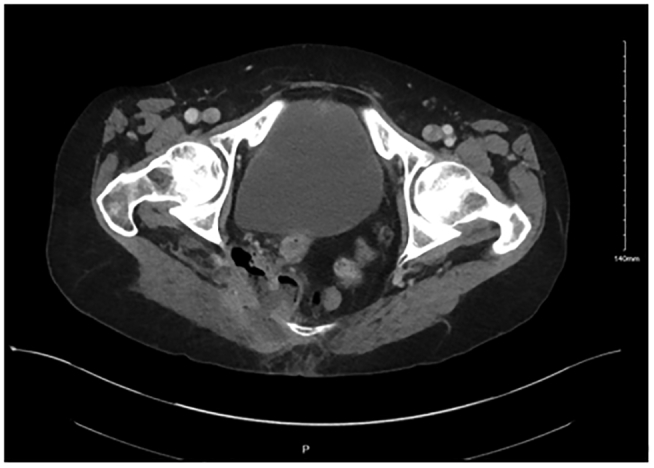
Pelvic abscess on hospital day 0 CT scan.
